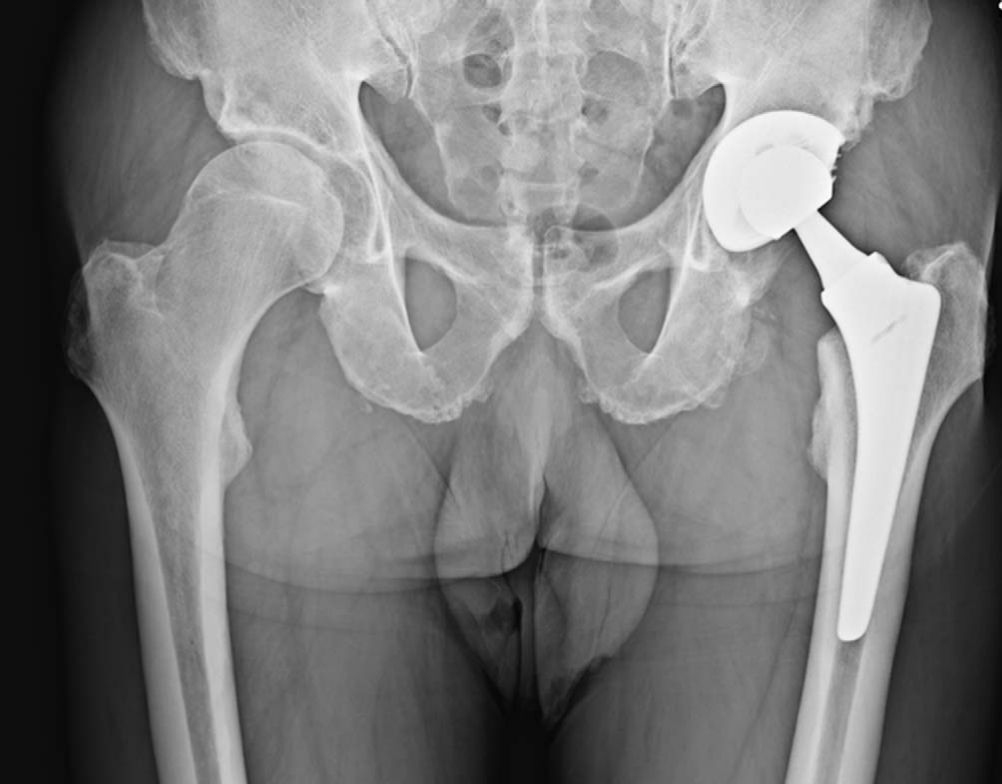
Anteroposterior pelvis X-ray showing CLS Brevius Kinectiv Stem 5 years after surgery with no signs of mobilization or osteolysis.
